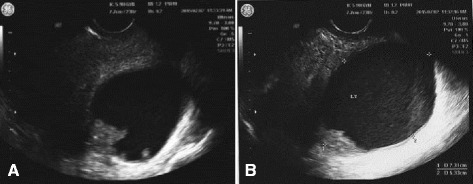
Transvaginal ultrasonography of the pelvis showing well-defined, bilateral, adnexal masses with papillary projections
